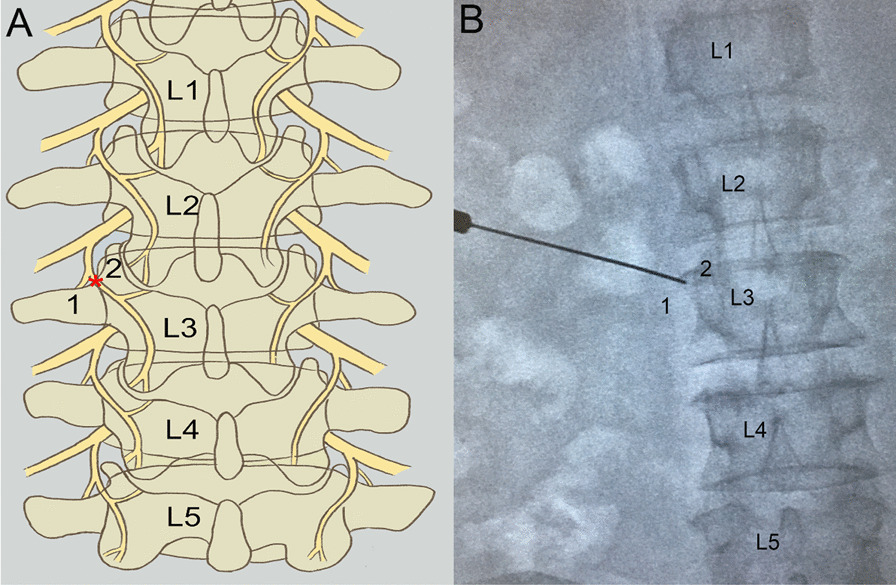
Anatomy of the posterior branch of the lumbar spinal nerve and X-ray guided radiofrequency needle insertion at target position. A: Branching of the posterior ramus of the lumbar spinal nerve at L3 (1: transverse process, 2: superior articular process, *: target position). B: Radiofrequency for the posterior ramus of the spinal nerve at L3. (Anterior–posterior fluoroscopy, 1: transverse process, 2: superior articular process)
